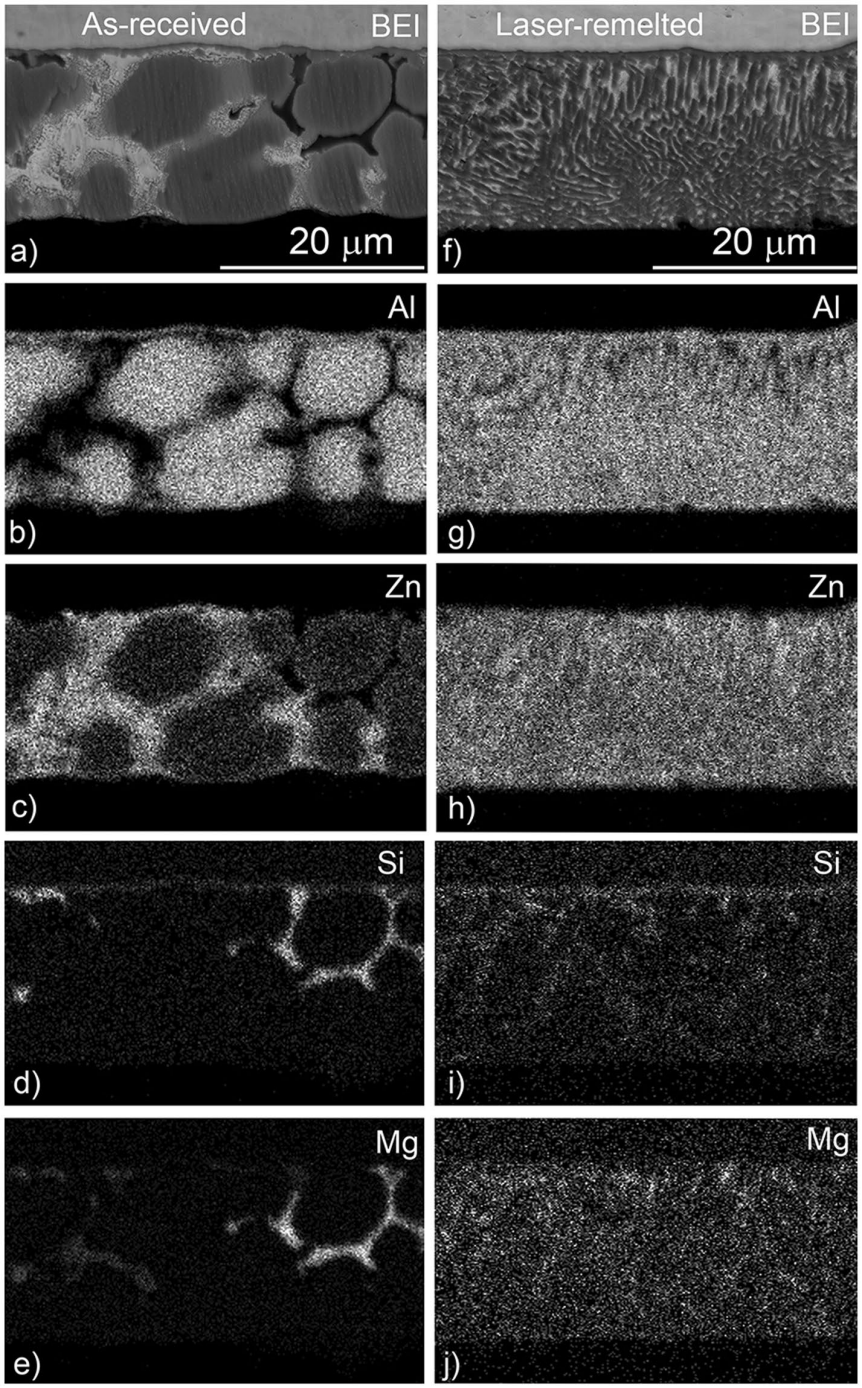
SEM/EDS X-ray mapping of Zn-55%Al-2%Mg-1.5%Si as-received (a–e) and laser remelted (f–j) coatings.
